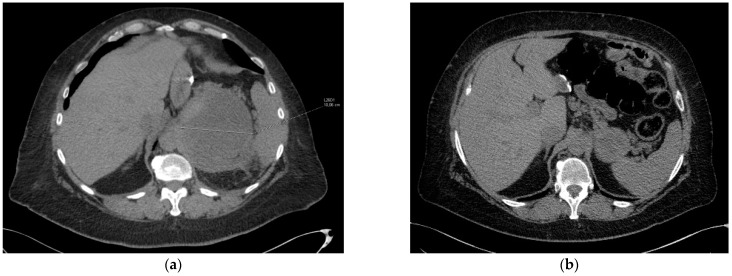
Partial response after BRAFi/MEKi treatment used in second-line. (a) CT scan from 28 December 2020, (b) CT scan from 1 April 2021.
